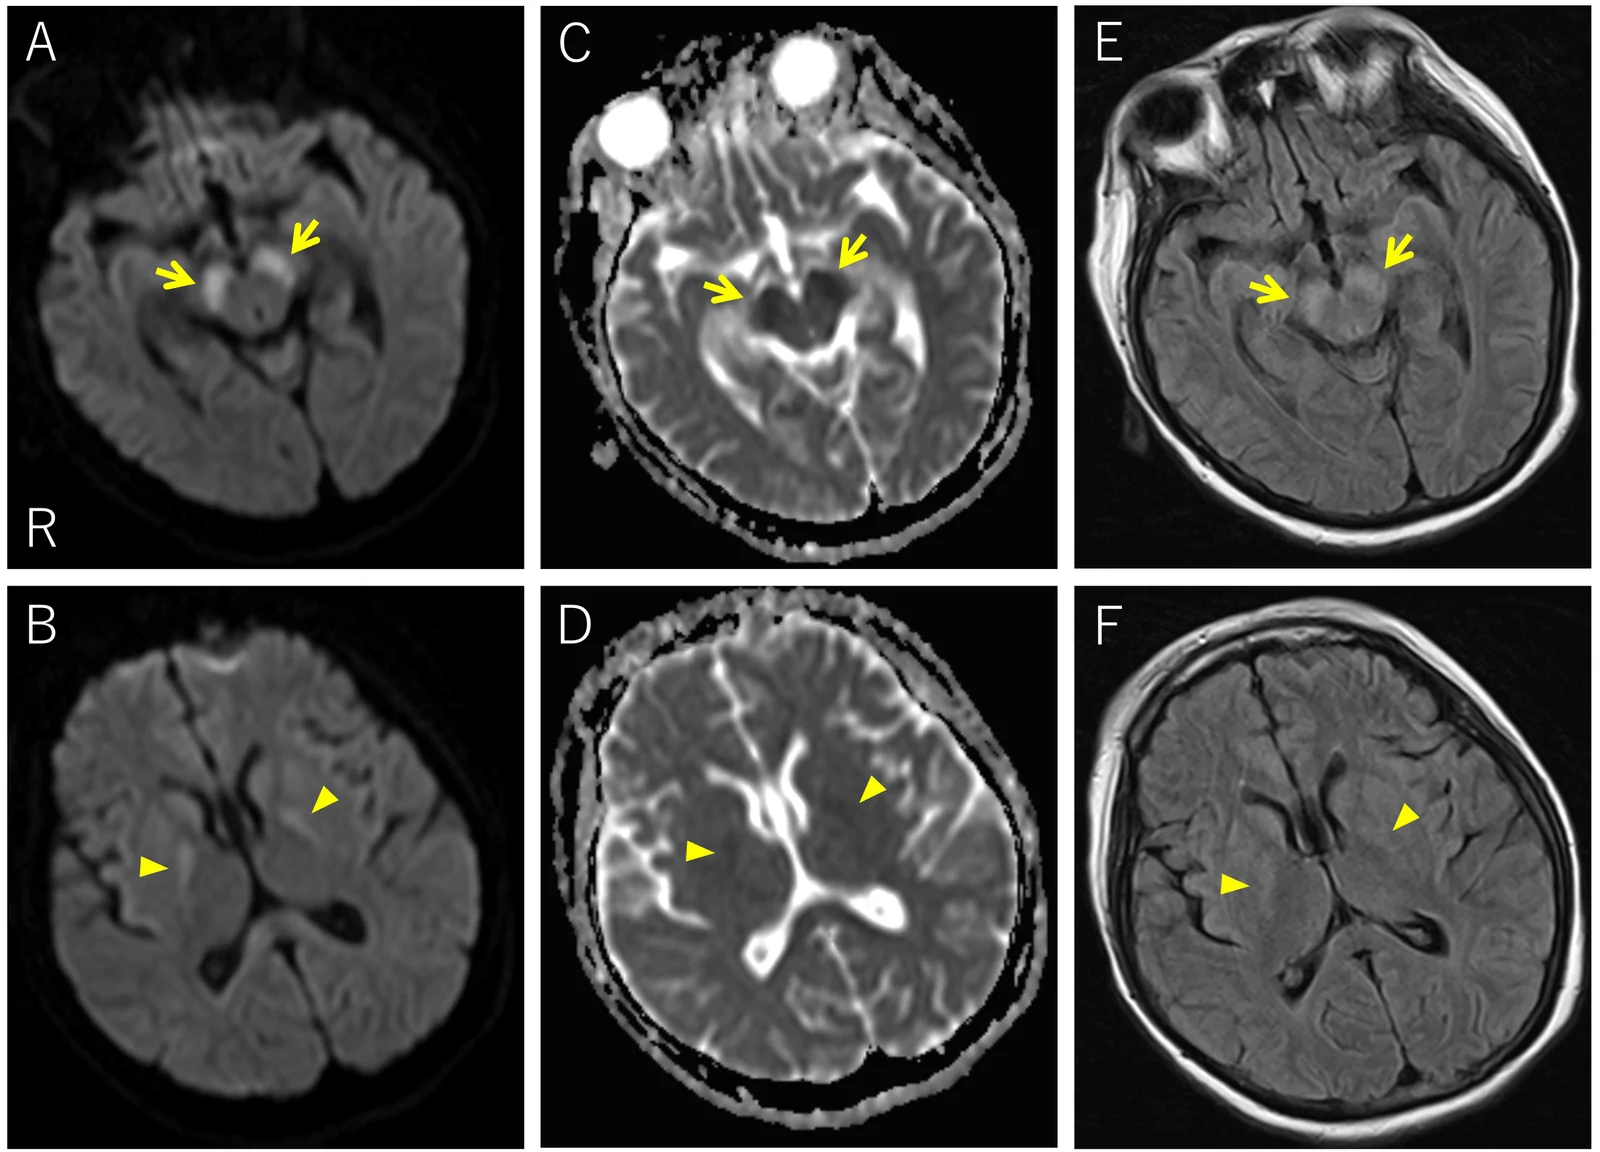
MRI findings on day 61 (A, B) Diffusion-weighted imaging (DWI) shows bilateral high signal intensities in the substantia nigra (A, yellow arrows) and the basal ganglia (B, yellow arrowheads). (C, D) The corresponding apparent diffusion coefficient (ADC) maps reveal decreased ADC values in the substantia nigra (C, yellow arrows) and the basal ganglia (D, yellow arrowheads), indicating restricted diffusion consistent with cytotoxic edema. (E, F) Fluid-attenuated inversion recovery imaging demonstrates mild hyperintensity in the same regions of the substantia nigra (E, yellow arrows) and the basal ganglia (F, yellow arrowheads) MRI: magnetic resonance imaging
